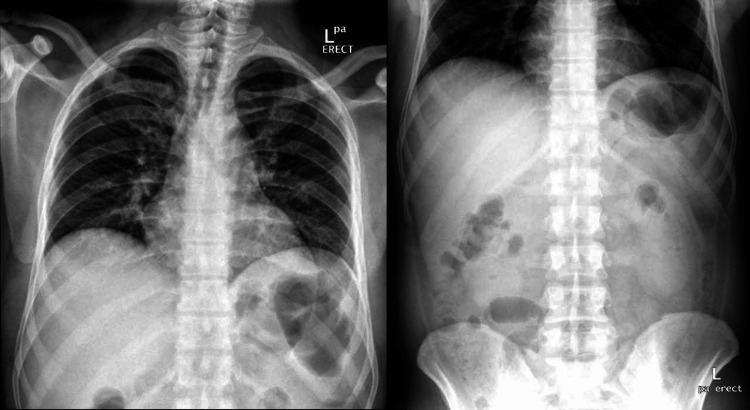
Chest X-ray, erect position (left): normal chest X-ray, no signs of pneumonia, or air-under diaphragm. Abdominal X-ray, erect position (right): normal bowel gas pattern. No evidence of bowel wall thickening. The vertebral column appears normal.
